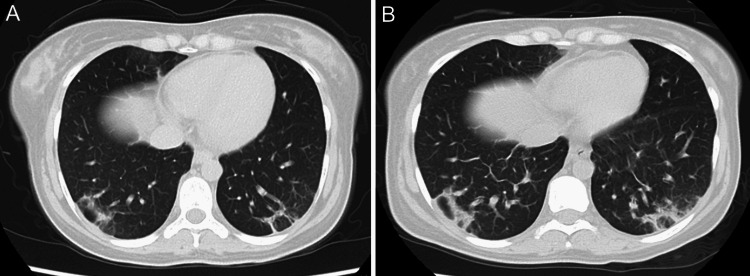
Plain chest CT scan image (A) At the first hospitalization, slight reticular shadows in the bilateral dorsal lower lung field were seen. (B) At the time of the second hospitalization, the reticular shadows slightly increased.
